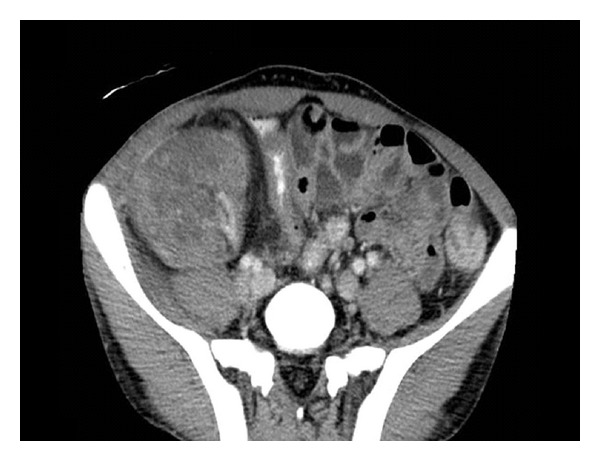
Computed tomography scan showing hyperdense areas in the renal allograft, in keeping with foci of parenchymal haemorrhage.
